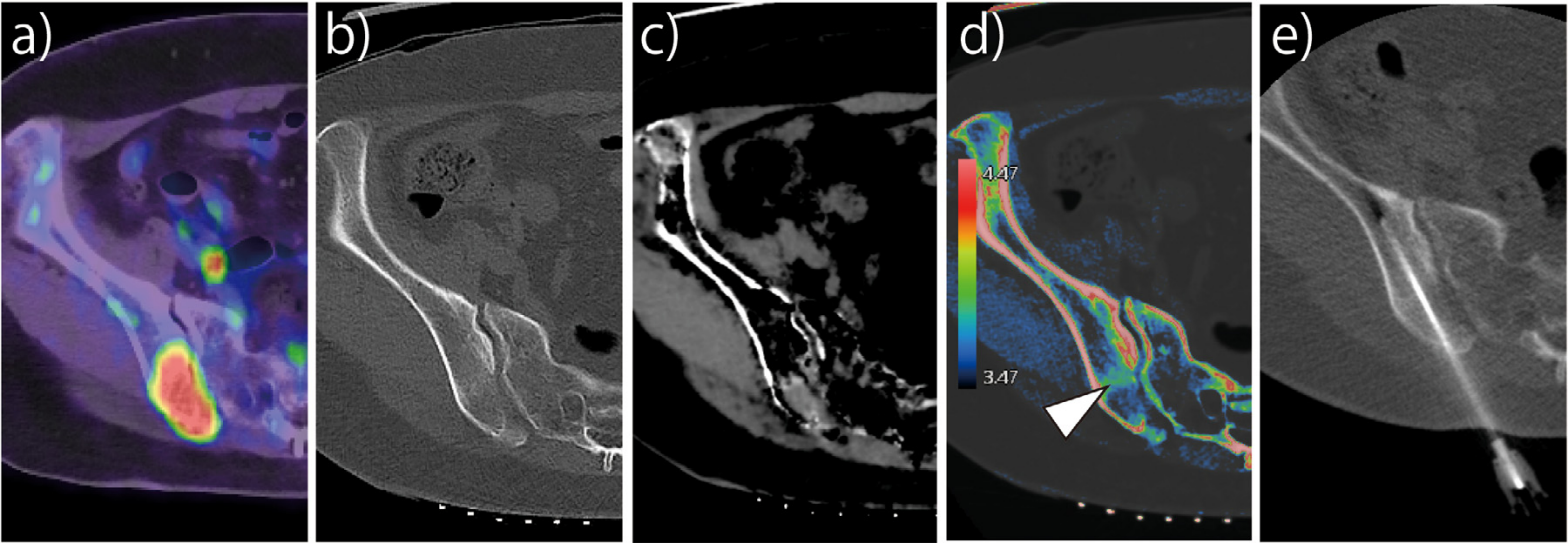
Images of the patient's right ilium. (a) An ^18^F-fluorodeoxyglucose positron emission tomography/computed tomography (FDG-PET/CT) image. The maximum standardized uptake value was 15.3. The patient's blood sugar at the time of imaging was 106 mg/dL. (b) A CT image to reveal bone condition. (c) Calcium suppression image (a virtual non-calcium technique). (d) Electron density map. *The high cellularity region showed high electron density (white arrowhead).* (e) A CT-guided bone biopsy image.
